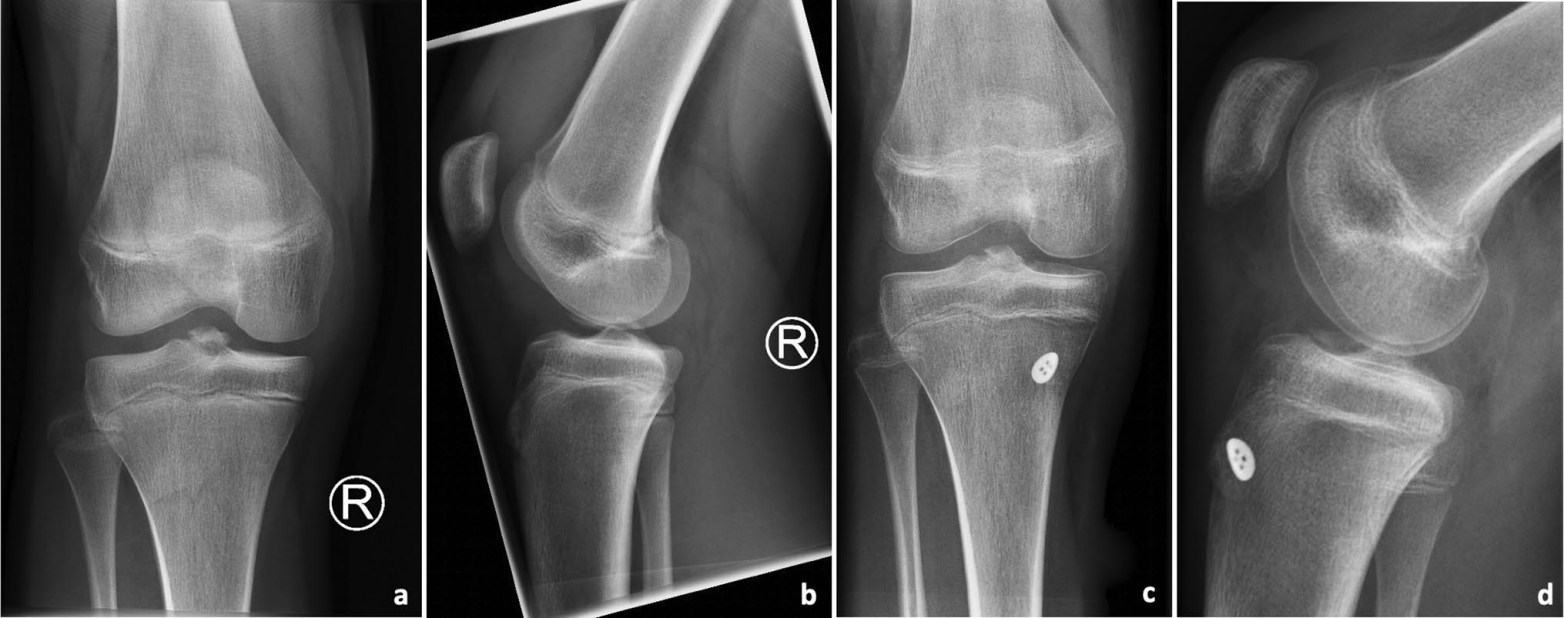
Standardized anterior–posterior and lateral radiographs in a right knee showing a tibial eminence avulsion fracture preoperatively (a–b) and on the first postoperative day after ARIF (c–d). For tibial fixation, an extracortical suture plate was used (c–d). *ARIF *arthroscopic reduction and internal fixation
